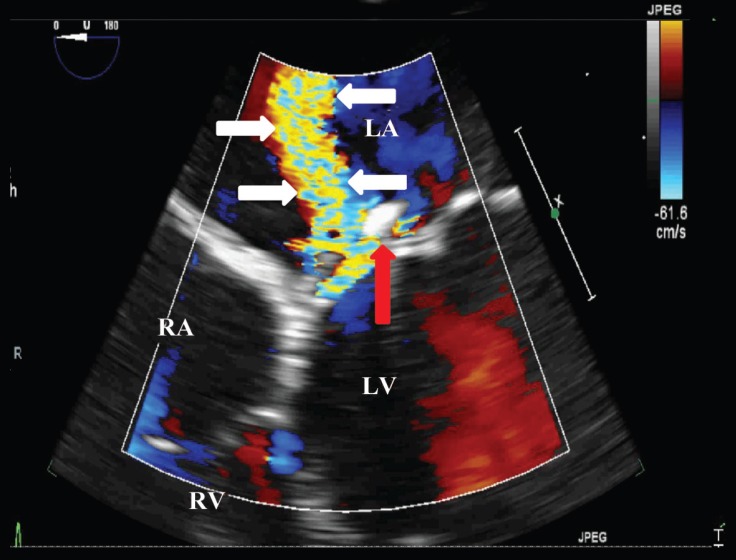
Transesophageal echocardiogram (4-chamber view), showing severe mitral regurgitation (white arrows) and another jet slightly toward the posterior wall of the left atrium (red arrow).
